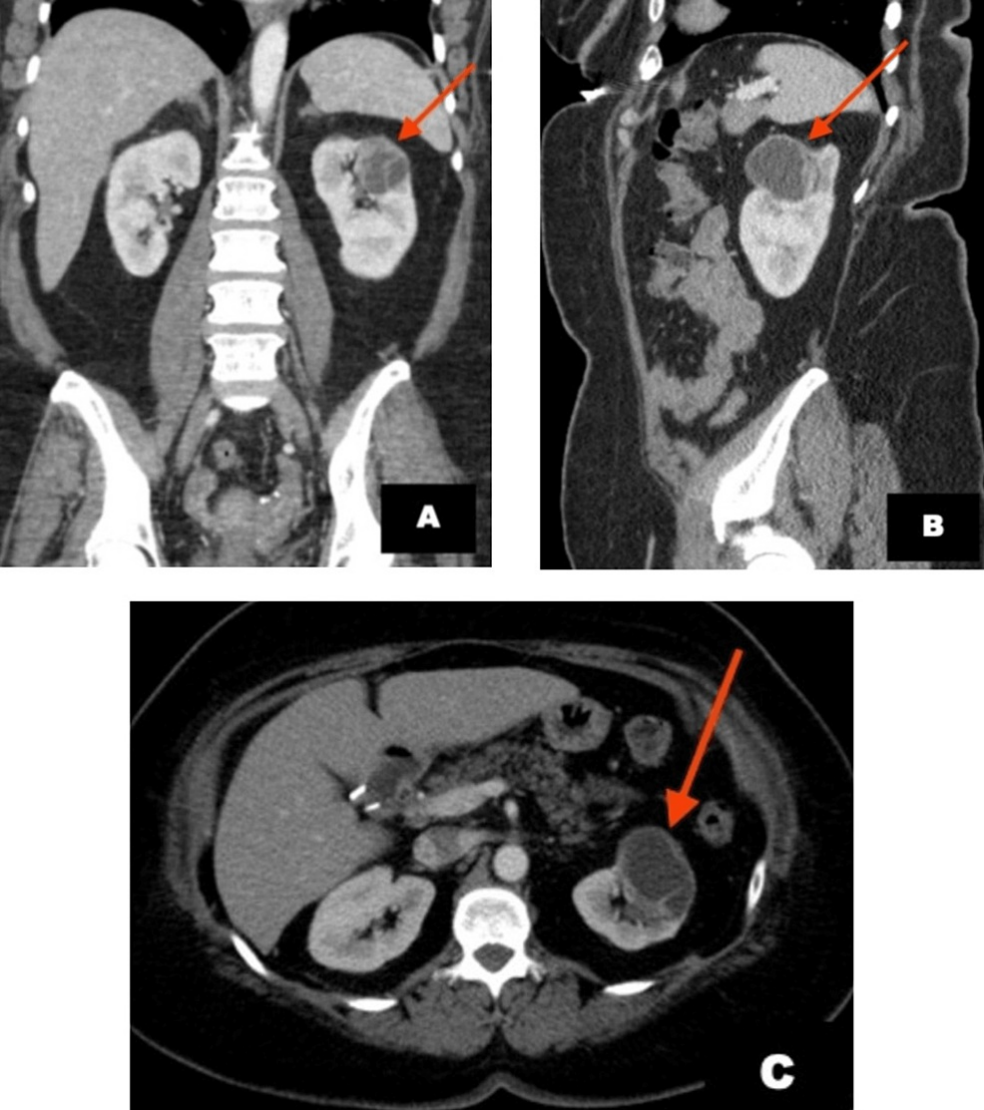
Lesion as observed on contrast-enhanced computed tomography scanning: (A) coronal view, (B) sagittal view, (C) axial view The study confirmed the presence of a partially exophytic, hypodense, cystic lesion in the upper pole of the left kidney with internal septations and no obvious perilesional fat stranding or edema.
